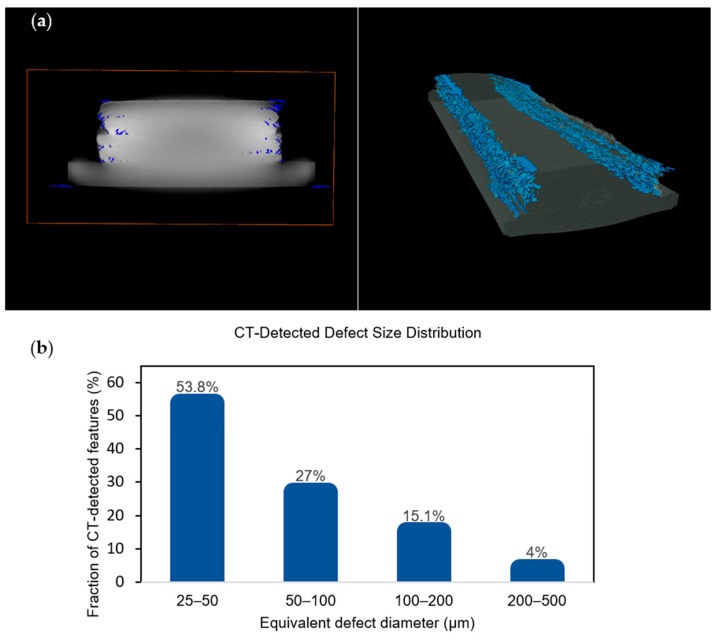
(a) X-ray CT scan of the AFSD-deposited Inconel 718 block showing a dense structure, with CT-detected defects highlighted in blue; (b) defect size distribution of the segmented CT-detected features based on equivalent diameter.
